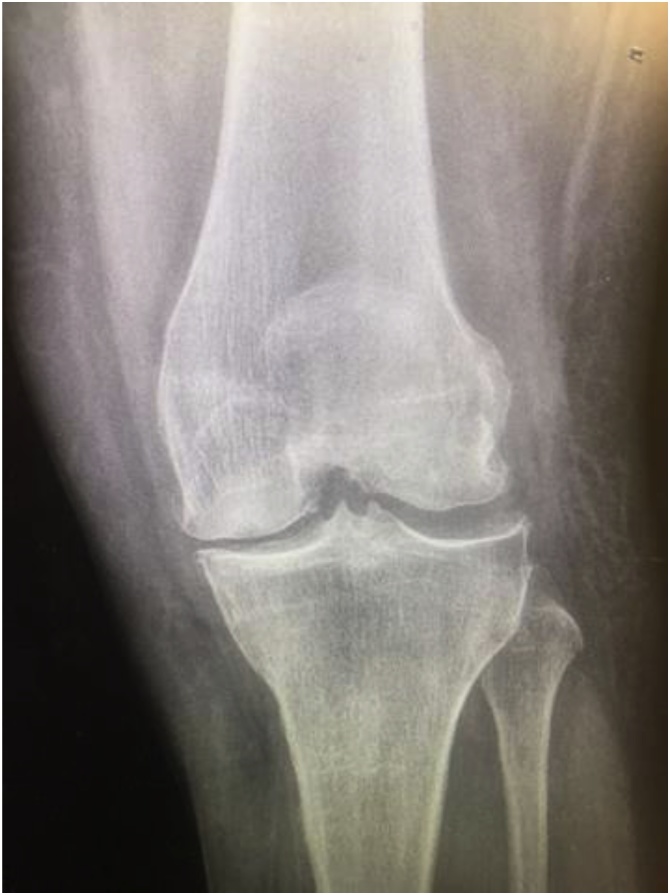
Left Knee AP Radiograph (Post Reduction).
